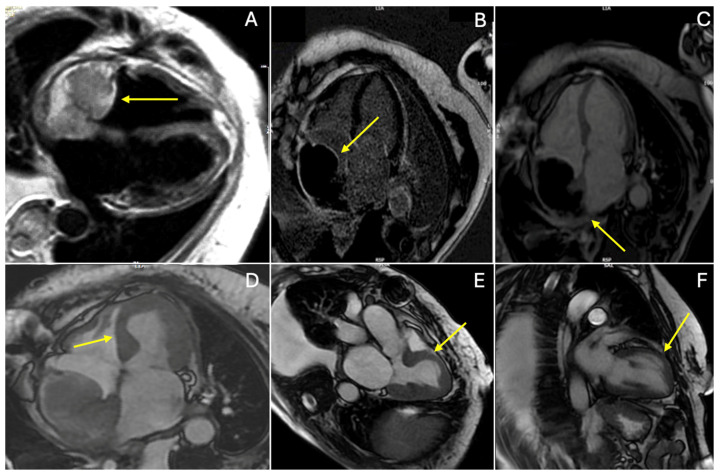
One month follow-up CMR showing RA mass (yellow arrows) appearing in (A) black blood T2-weighted image with heterogenous signal; (B) late gadolinium enhancement (LGE), showing no contrast uptake in the mass; (C) early gadolinium enhancement (EGE), showing no contrast uptake in the mass. Note the hyperintense signal in the black blood T2-weighted image (A) in the medial segment of the septum due to edema as part of Takotsubo cardiomyopathy; CMR steady-state free precision cine images in long-axis view showing wall motion abnormalities due to Takotsubo cardiomyopathy (D) in four-chamber dyskinesia of the medial segment of septum, (E) three-chamber dyskinesia of medial segments of anterior septal wall, and (F) two-chamber dyskinesia of medial segments of anterior wall.
